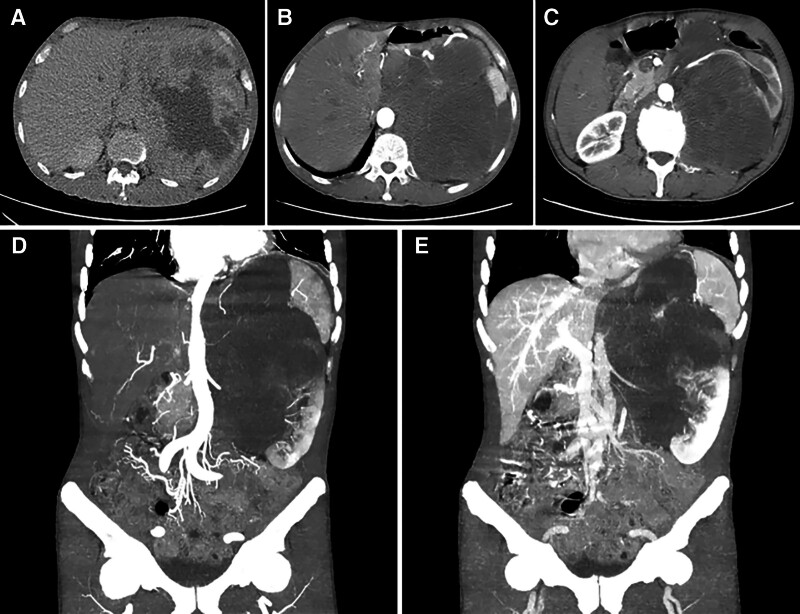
CT scan of the retroperitoneal tumor. (A) There was extensive necrosis in the tumor. (B) The abdominal aorta was compressed to the right of the spine. (C) The left renal artery passed through the tumor. (D) The tumor pushed bowels into the right abdomen and pelvis. (E) The tumor significantly invaded the left kidney. The splenic vessels were wrapped in the tumor.
